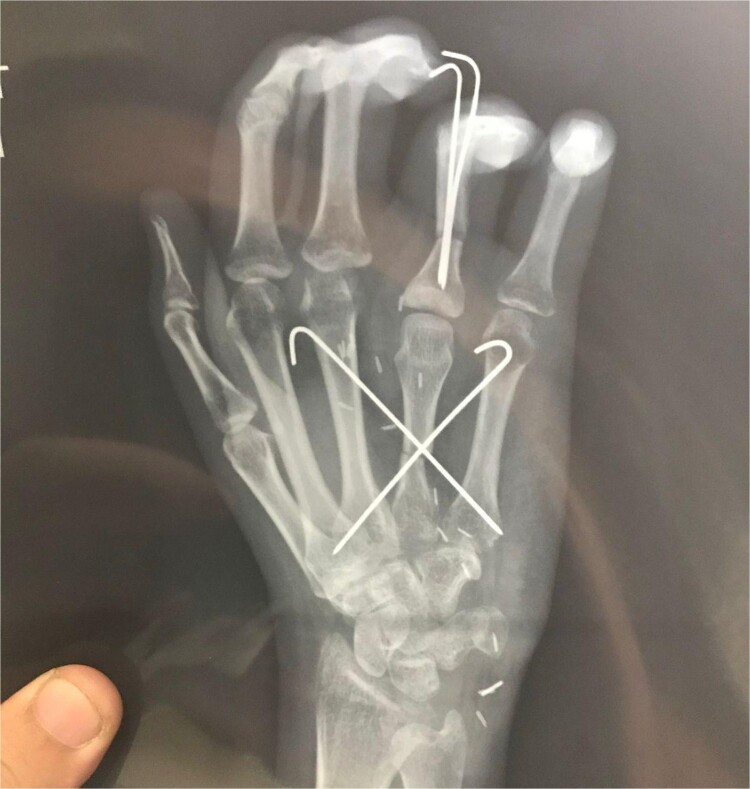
Post-operative X-ray at 3 weeks.
